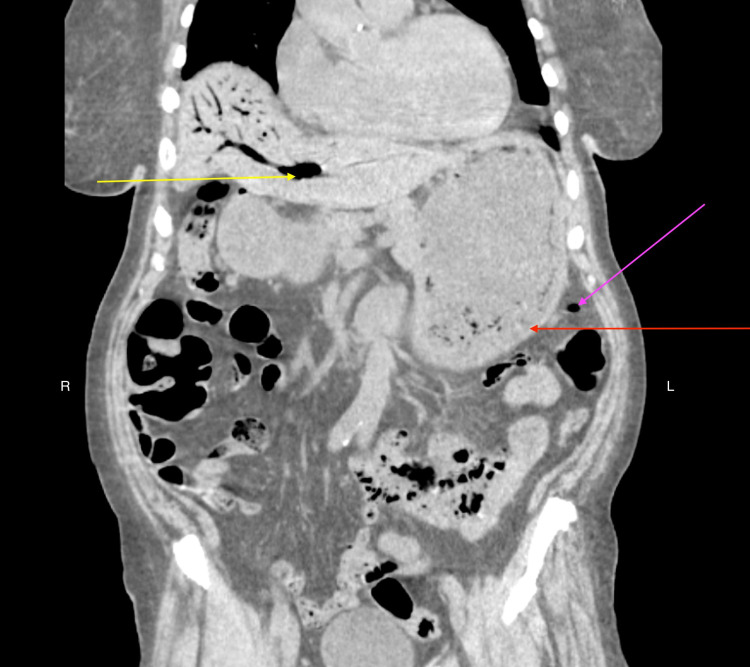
CT scan of abdomen without contrast (coronal view) demonstrating thickened gastric wall with gas bubbles (red arrow), gas in portal venous system - main portal vein, right portal vein, and intra-hepatic veins (yellow arrow), and gas in peri-gastric vein (pink arrow).
